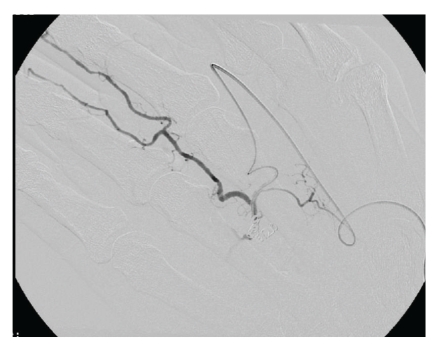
Postcoiling CTA confirming no flow in neck of pseudoaneurysm.
